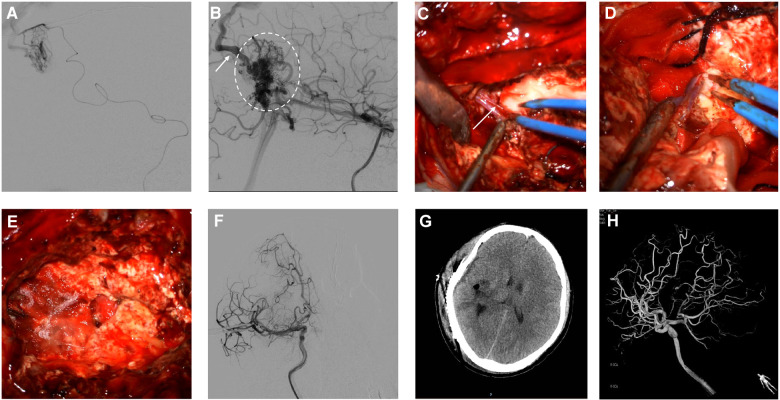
Intraoperative angiography demonstrates subsequent injection of Onyx18 with obliteration of the AVM (A). Lateral view of right ICA angiogram demonstrates the presence of residual arteriovenous malformation (dashed oval) with an early draining vein (arrow) (B). The feeding artery arteries and main draining veins were isolated and resected (C,D, arrows). Intra-operative image shows lesion excised during surgery (E). Postoperative anteroposterior projection angiograms following resection demonstrate the obliteration of the AVM (F). Postoperative Contrast CT shows no obvious significant ischemic or hemorrhage changes (G). Follow-up 3D DSA shows the complete disappearance of AVM (H).
